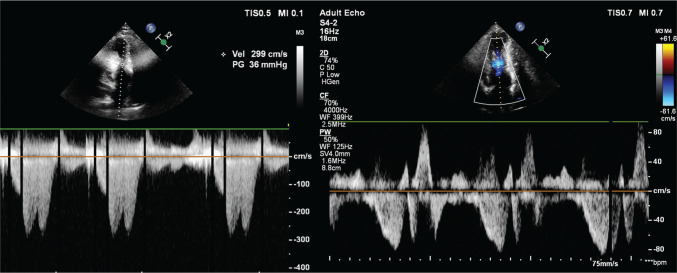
The control echocardiographic examination shows a reduction in the interventricular gradient from 36 to 6 mmHg.
